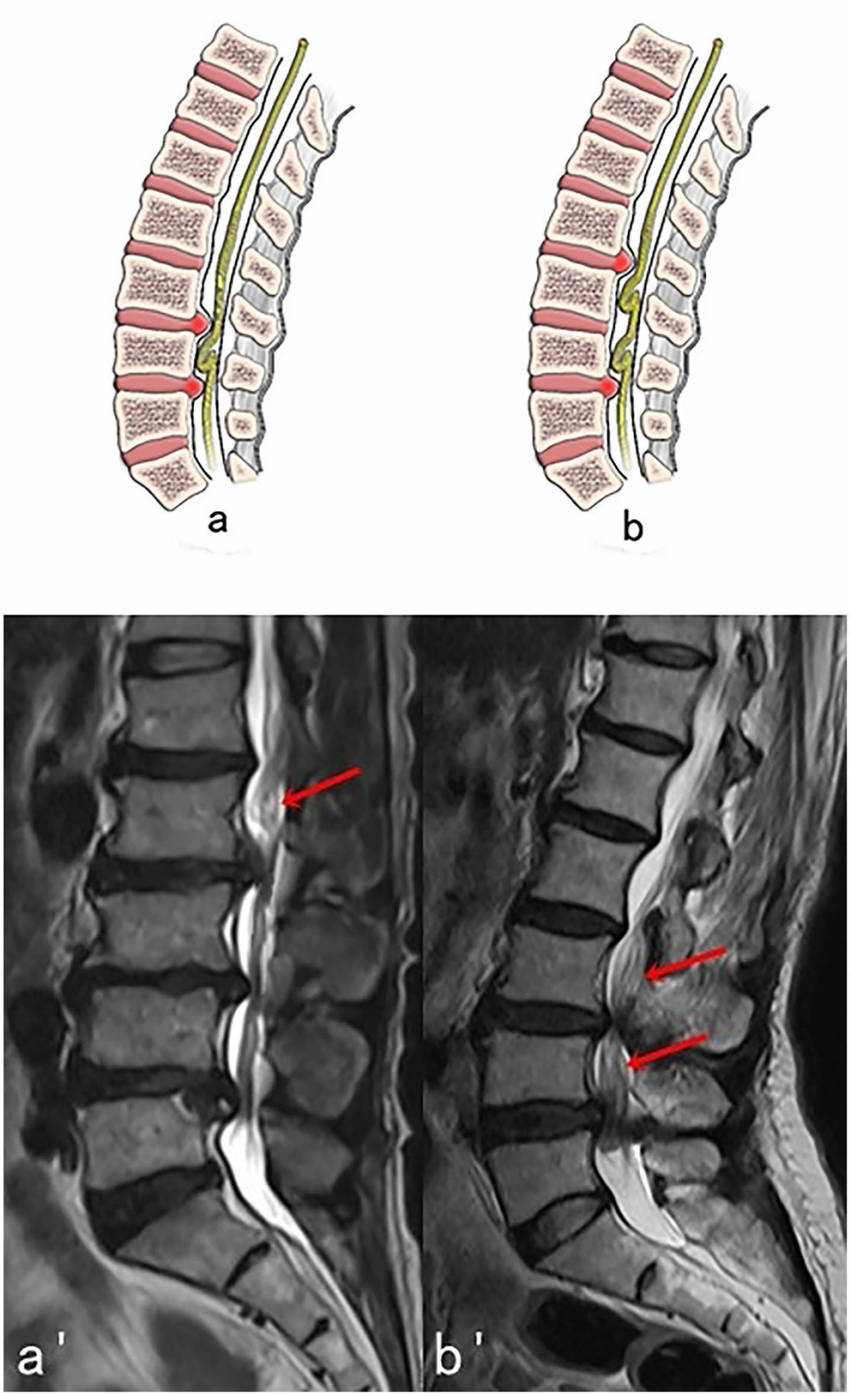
Two possible subtypes of Intermediate RNRs (RNRs which are between the stenosis) with corresponding MRI images. (A,A′) One segment of RNRs (red arrow) are between the stenosis. (B,B′) Two segments of RNRs (red arrows) are between the stenosis.
